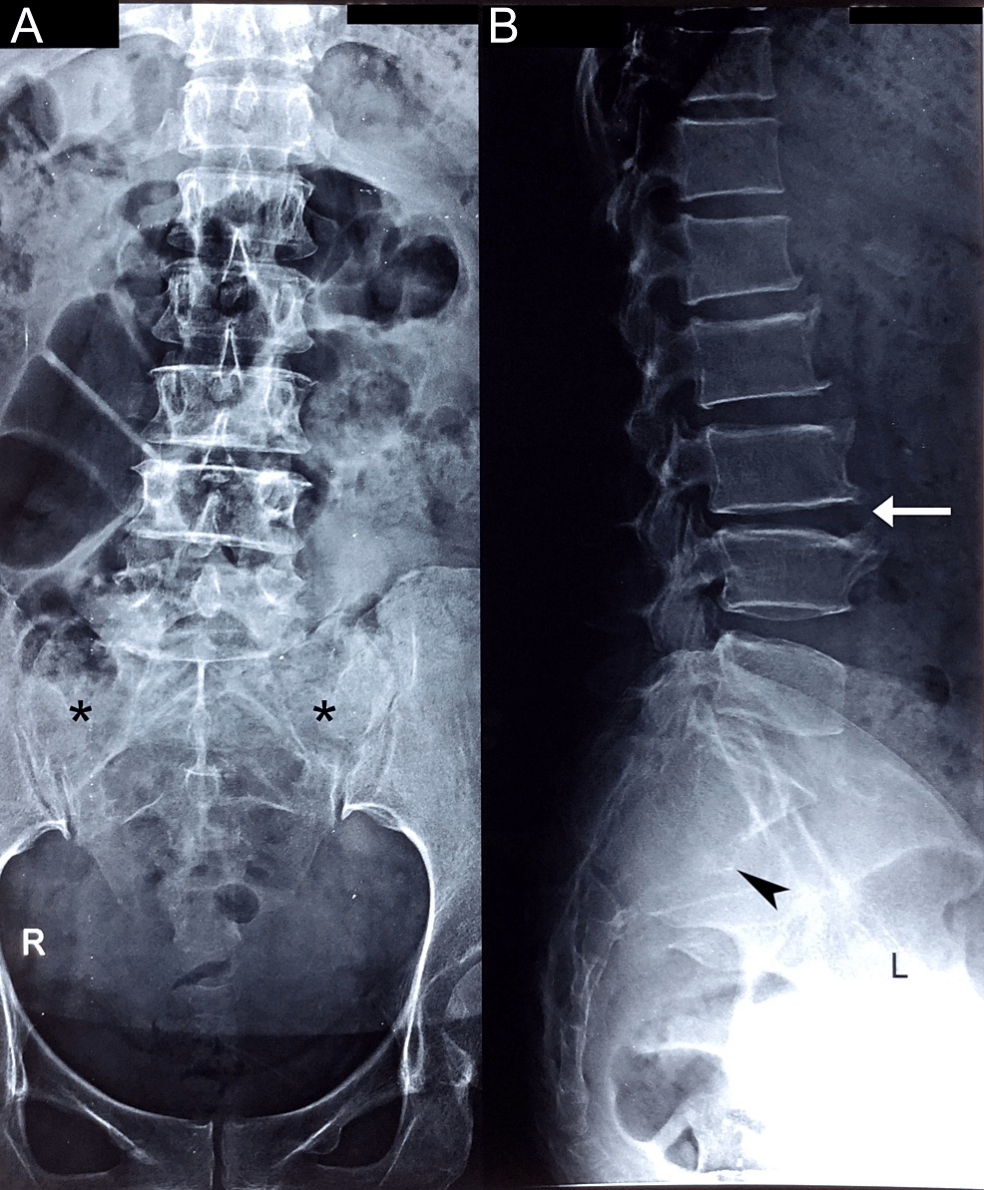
Lumbar radiographs, anteroposterior view (A) and lateral view (B). These radiographs were originally interpreted as only showing marginal osteophytes and disc space narrowing at L3-L4, which is best seen on the lateral view (arrow). However, after advanced imaging, it was apparent that there were subtle signs of abnormality that were not identified earlier, including a slight lucency of the sacral alae (*) and disruption of the anterior cortex of the sacrum (arrowhead). These findings are partially obscured by bowel gas in image A and overlapping anatomy of the pelvis in image B.
